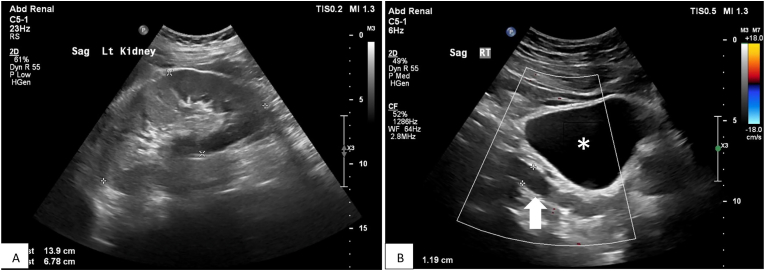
(A) ultrasound confirms the known finding of an absent right kidney and shows a hypertrophic left kidney that measured 13.9 cm in length. (B) ultrasound shows a tubular anechoic fluid filled structure (arrow) posterior lateral to the right side of the bladder (*).
